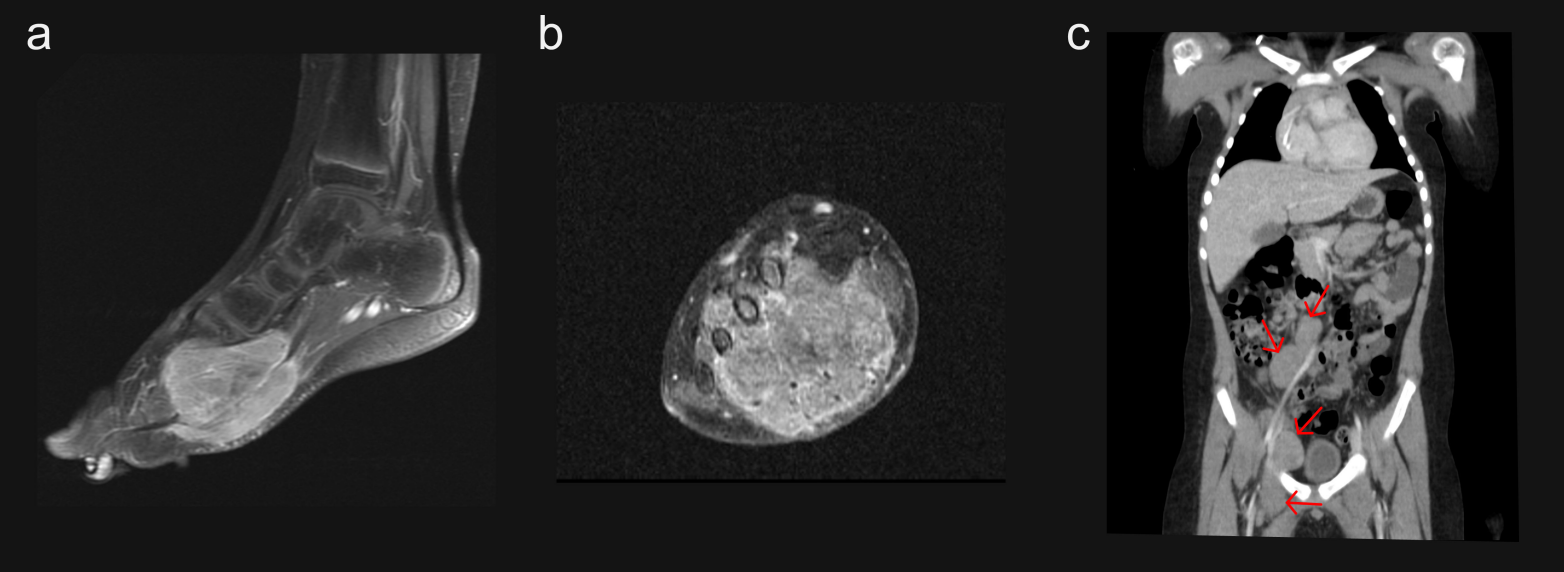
Primary tumors at initial diagnosis. (a) MRI sagittal plane of the right foot; (b) MRI coronal plane of the right foot; (c) Abdominal CT coronal plane showing inguinal and retroperitoneal malignant lymph nodes. CT, computed tomography; MRI, magnetic resonance imaging.
